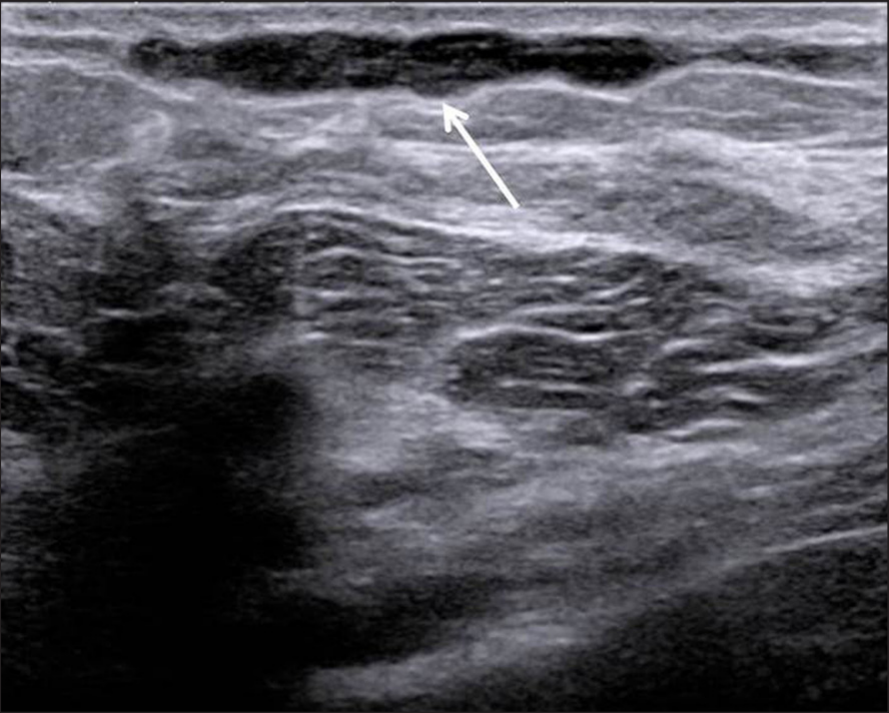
2D ultrasound: hypoechogenic subcutaneous and uncompressible linear structure.
